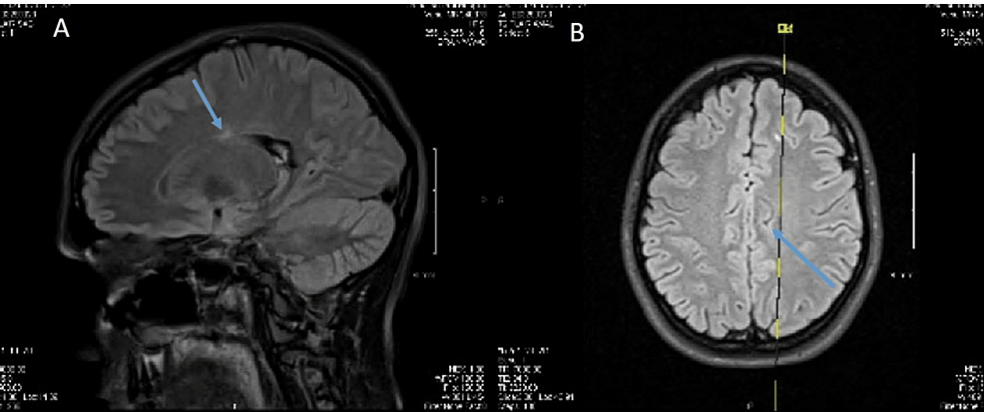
A (Sagittal) and B (Transverse) MRI demonstrating numerous small, non-enhancing periventricular and juxtacortical T2 hyperintensities (arrow).
